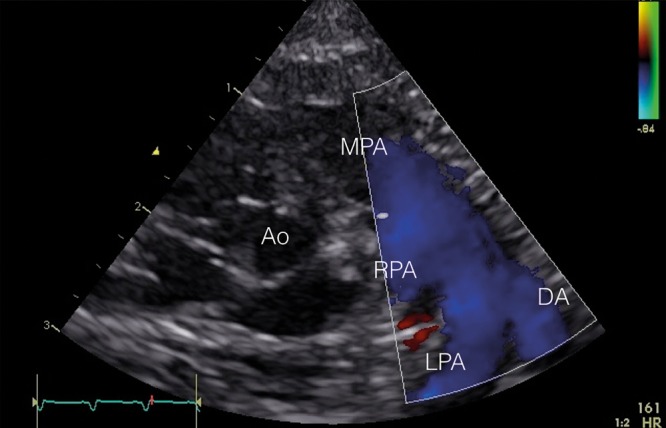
Transductal right-to-left shunting. In the parasternal short axis view, the main pulmonary artery (MPA) is shown with the right pulmonary artery (RPA), the left pulmonary artery (LPA), and the ductus arteriosus (DA) with a clear right-to-left shunt (blue in color Doppler)
